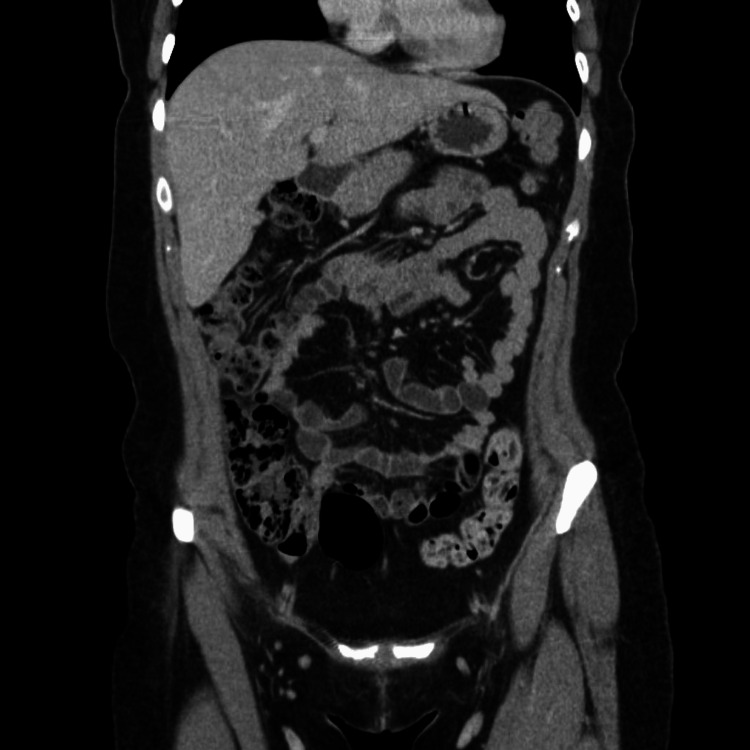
Coronal view of abdominal and pelvic CT with contrast (reported as a non-visualized gallbladder). There appears to be no sign of previous surgical intervention such as surgical clips. Initial review led to a left-sided gallbladder as a differential, but when correlated with a cross-sectional view (Figure 5), this appears to be the first part of the duodenum. CT: computed tomography.
